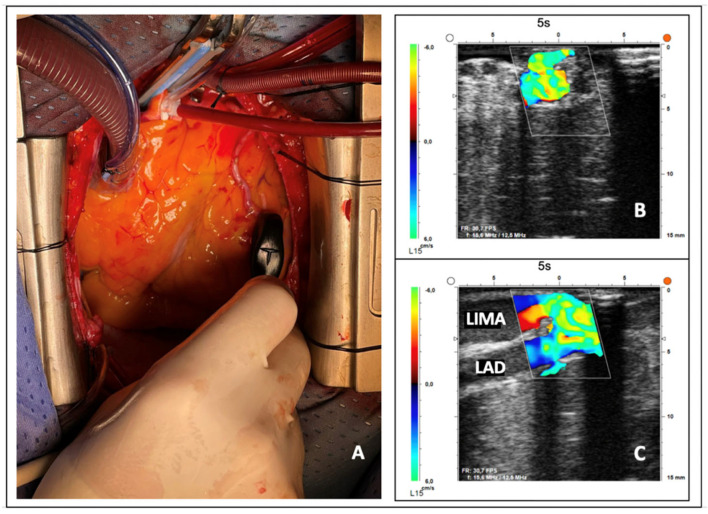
High-resolution epicardial ultrasound (HRUS) scoring system. After completion of each distal anastomosis, the resident performed HRUS acquisition (A). The objective was to obtain, within a 1−min time limit, both a short-axis (B) and a long-axis (C) 2D and color-flow image. The scoring system followed these criteria: short−axis acquisition within 60 s = 1 point; adequate short-axis color−flow acquisition = 1 point; long-axis acquisition within 60 s = 1 point; adequate long-axis color-flow acquisition = 1 point. The maximum achievable score was 4, and the minimum was 0. After image acquisition, the resident was required to assess the quality of the anastomosis. In the event of a misjudgment (i.e., a patent anastomosis incorrectly classified as inadequate, or vice versa), the total score for that graft (HRUS + TTFM) was reset to zero. A projection was considered adequate when the following criteria were met: for the short−axis view, perpendicular alignment to the graft axis with clear visualization of the lateral borders, central lumen, and posterior wall of the anastomosis; for the long−axis view, correct longitudinal alignment showing the heel, toe, and receiving coronary vessel, both proximal and distal to the anastomosis. Abbreviations: LIMA = left internal mammary artery; LAD = left anterior descending artery.
